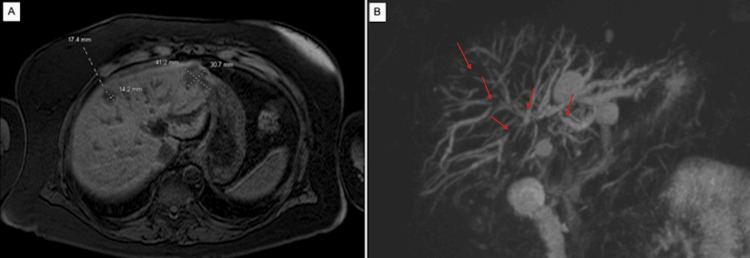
MRI (A) and MRCP (B) performed during the second hospital admission demonstrating hepatic lesions and intrahepatic biliary strictures, respectively. Red arrows indicate representative intrahepatic biliary strictures with associated upstream ductal dilation. MRI: Magnetic Resonance Imaging; MRCP: Magnetic Resonance Cholangiopancreatography
